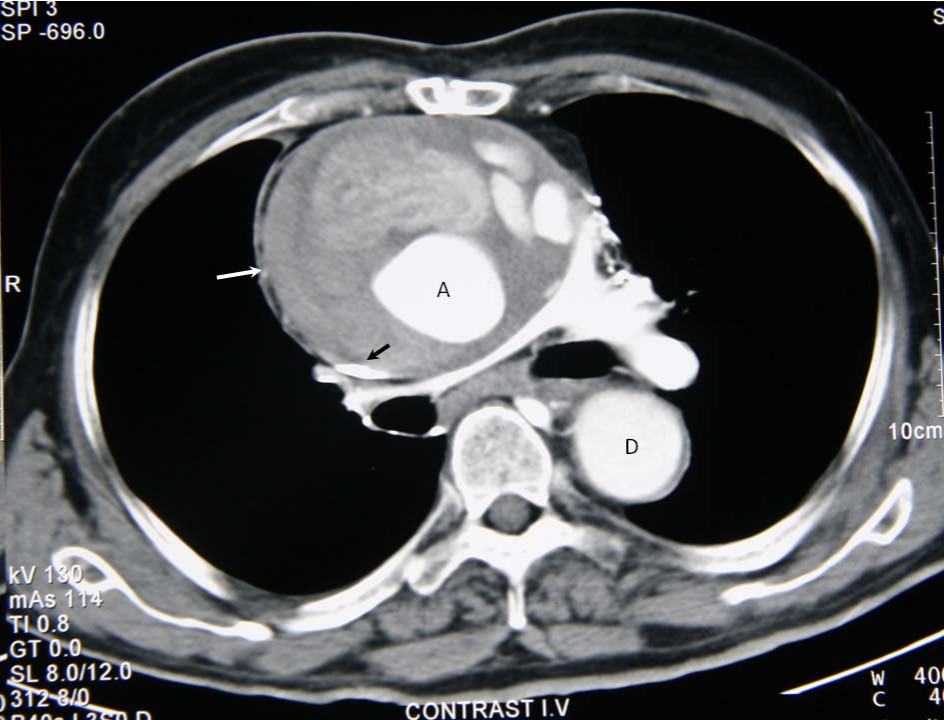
Contrast CT scan. Contrast computed tomography of the superior mediastinum showing (white arrow) the pseudoaneurysm of the aorta causing compression of superior vena cava (black arrow). (A denotes ascending aorta, D denotes descending aorta).
